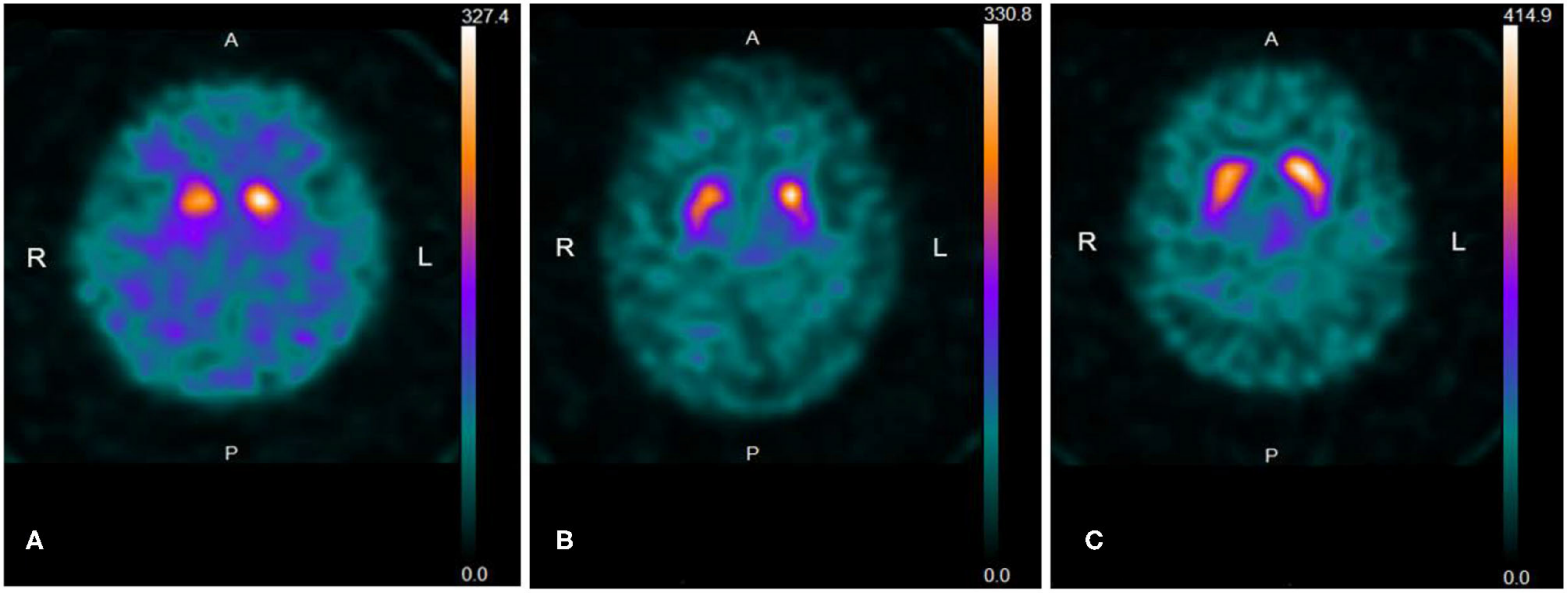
(A) Representative ^123^I-FP-CIT SPECT (DaTscan) image of an “egg shape” pattern. The patient is a 78-year-old woman with a UPDRS-III score of 4. Uptake of DaTscan is almost absent in both putamina and normal or almost normal in caudate nuclei, showing an “egg shape” pattern. SBR is bilaterally reduced (R: 1.61; L: 1.96, Avg; 1.79) and AI is higher (19.6%) than the normal range (<10.9%). SBR: specific binding ratio, AI: asymmetry index. (B) Representative DaTscan image of a “mixed type” pattern. The patient is an 85-year-old man with a UPDRS-III score of 4. A DaTscan image shows normal or almost normal tracer uptake in bilateral caudate nuclei with asymmetrical tracer uptake reduction in the putamen of the left side by visual assessment, showing a “mixed type” pattern. SBR is bilaterally reduced (R: 2.60; L: 2.95, Avg: 2.77) and AI is increased (12.7%) (normal range: <11.05%). (C) The patient is an 80-year-old woman with a UPDRS-III score of 4. The pattern of uptake of DaTscan is almost normal by visual assessment. However, SBR is reduced in the striata with right-side predominance (R: 3.09; L: 3.83, Avg: 3.46), and AI is increased (21.3%) (normal range: <11.05%).
